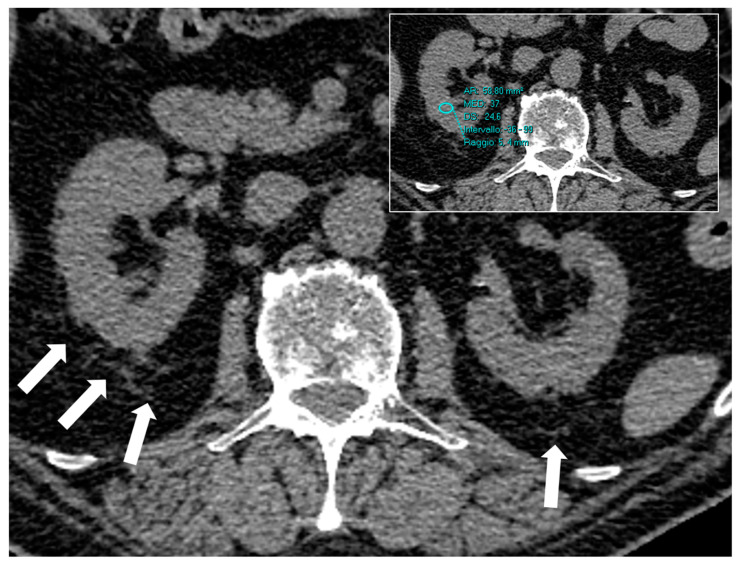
Axial non-enhanced CT of a 65-year-old admitted with COVID-19. CT images show a typical case of perinephric fat stranding (arrows). Renal parenchyma attenuation was 37 HU in this case. An ROI area, as an example, is shown in the box. This patient died in hospital.
